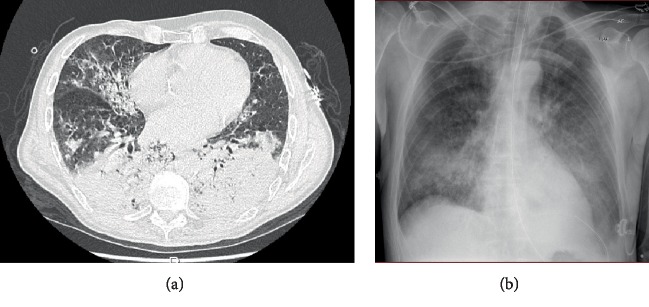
Computed tomography of lungs at admission (a) and chest X-ray immediately after intubation (b) showing massive bilateral pneumonia.
